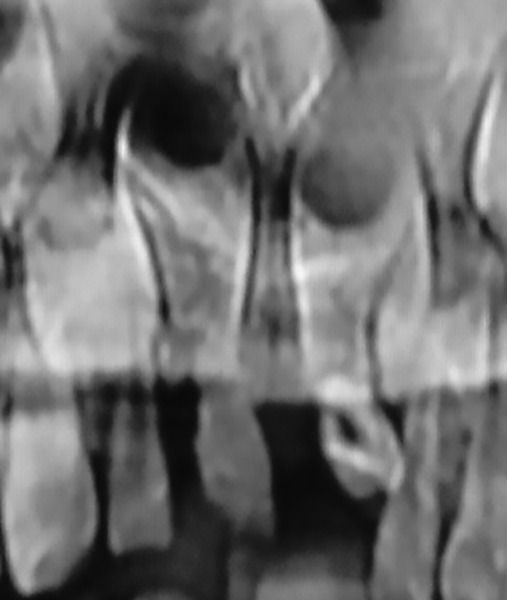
Intraoral periapical radiograph irt 51, 61
